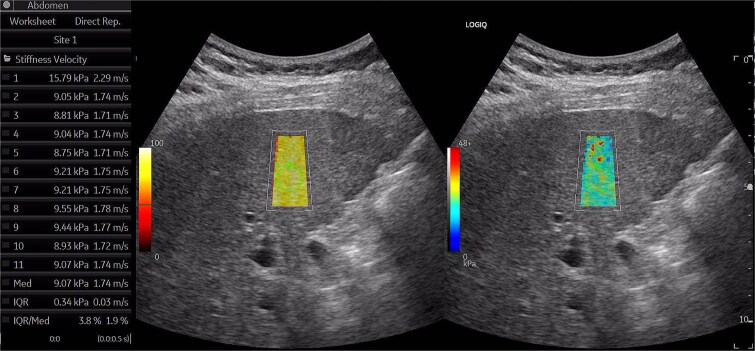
Ultrasound images show a slightly coarse liver texture and a mildly obtuse liver angle. Liver elasticity, assessed with shear wave Elastography on the GE LOGIQ FORTIS machine, corresponds to F2 according to the Metavir classification, with a median velocity of approximately 1.75 m/s. the liver stiffness classification table for the GE LOGIQ FORTIS machine is as follows: F0 (<1.35 m/s), F1 (1.35–1.65 m/s), F2 (1.66–1.76 m/s), F3 (1.77–1.99 m/s), F4 (>1.99 m/s).
